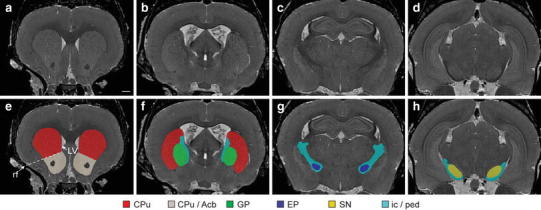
Anatomical segmentation of structural MRI images. Selected coronal slices from high-resolution *T*
